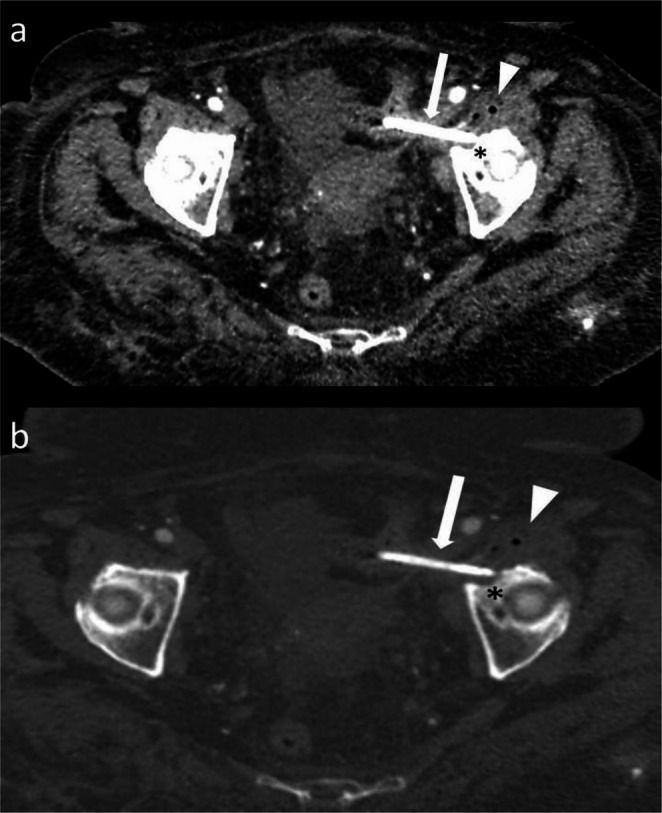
Axial contrast-enhanced CT of the pelvis in soft tissue window (a) and bone window (b). A biliary stent perforating the sigmoid colon wall (arrow), a left iliopsoas muscle abscess (arrowhead), and focal erosion on the acetabulum (*) are seen. Peripherally, sclerotic changes extending toward the anterior acetabular roof are observed in the ilium, along with cortical irregularities and small erosions with sclerotic borders
